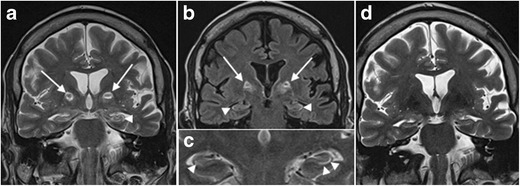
Presumed carbon monoxide poisoning. A 49-year-old man was found comatose and with an alcohol intoxication in his garage next to his car, which was not running. The patient received an MRI 14 days after admittance to the hospital. Coronal T2-weighted (a,c) and FLAIR images (c) showed T2-hyperintense lesions with central iso- to hypo-intense areas in both pallidi (*white arrows*) as well as T2 hyper-intensity of both hippocampi from head to tail involving the CA2 sector on the right and the CA1 and CA2 sectors on the left (*white arrowheads*). On follow-up MRI performed 4 months later, these abnormalities had disappeared (d). The patient suffered from amnesia and the details of what happened exactly remain a mystery to this date. The patient was suicidal and the abnormalities in the pallidi are very suggestive for carbon monoxide poisoning, however, with the hippocampal abnormalities reflecting damage to the especially vulnerable Sommers CA2 sector of the hippocampi
